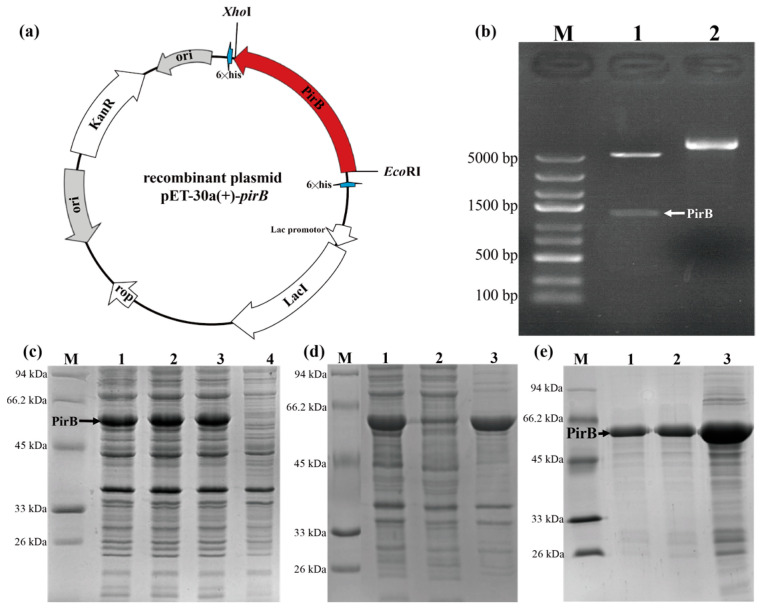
Verification and purification of recombinant protein PirB. (a) Recombinant plasmid map of pET-30a(+)-*pirB*. (b) Identification results of PirB recombinant plasmid. M: DNA Marker DL 5000; 1: Plasmid digested by *Eco*RI and *Xho*I; 2: Plasmid DNA. (c) The small-amount expression gel map of the PirB protein. M: Marker; 1–3: IPTG-induced recombinant BL21 expression bacteria; 4: Recombinant BL21 expression bacteria. (d) Bulk expression gel map of PirB protein. M: Marker; 1: Ultrasonically crushed whole bacteria; 2: Ultrasonic crushing with supernatant; 3: Sedimentation after ultrasonic crushing. (e) PirB protein mass purification and expression gel map. M: Marker; 1: 10-fold dilution of purified protein; 2: 5-fold dilution of purified protein; 3: Original sample of purified protein.
